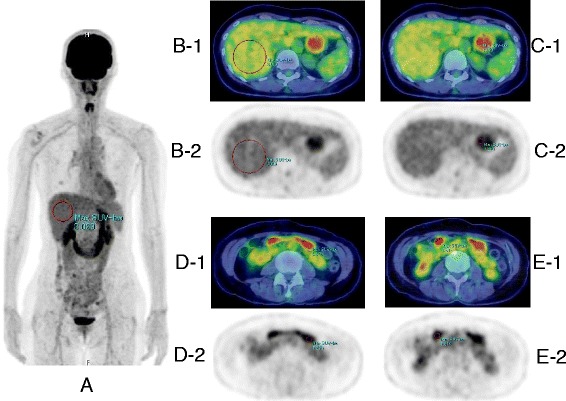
The methods for measuring ^18^F-FDG uptake of the stomach and the liver. VOIs of 3D sphere were placed at the fornix, corpus and antrum of the stomach and the liver in each subject. SUVmax (shown as Max SUV-bw on MIP, fusion images and PET images) and SUVmean were determined in each VOI in the stomach, and SUVmean liver was determined in liver VOI. a A MIP image of a subject with *H. pylori* infection, VOI was placed to avoid the area just blow the diaphragm for preventing the motion blurring artifact. The VOIs were placed by monitoring both PET/CT fusion images and PET images. b-1, 2 VOI of the liver. c1, 2 VOI of gastric fornix. d-1, 2 VOI of gastric corpus. e-1, 2 VOI of gastric antrum
